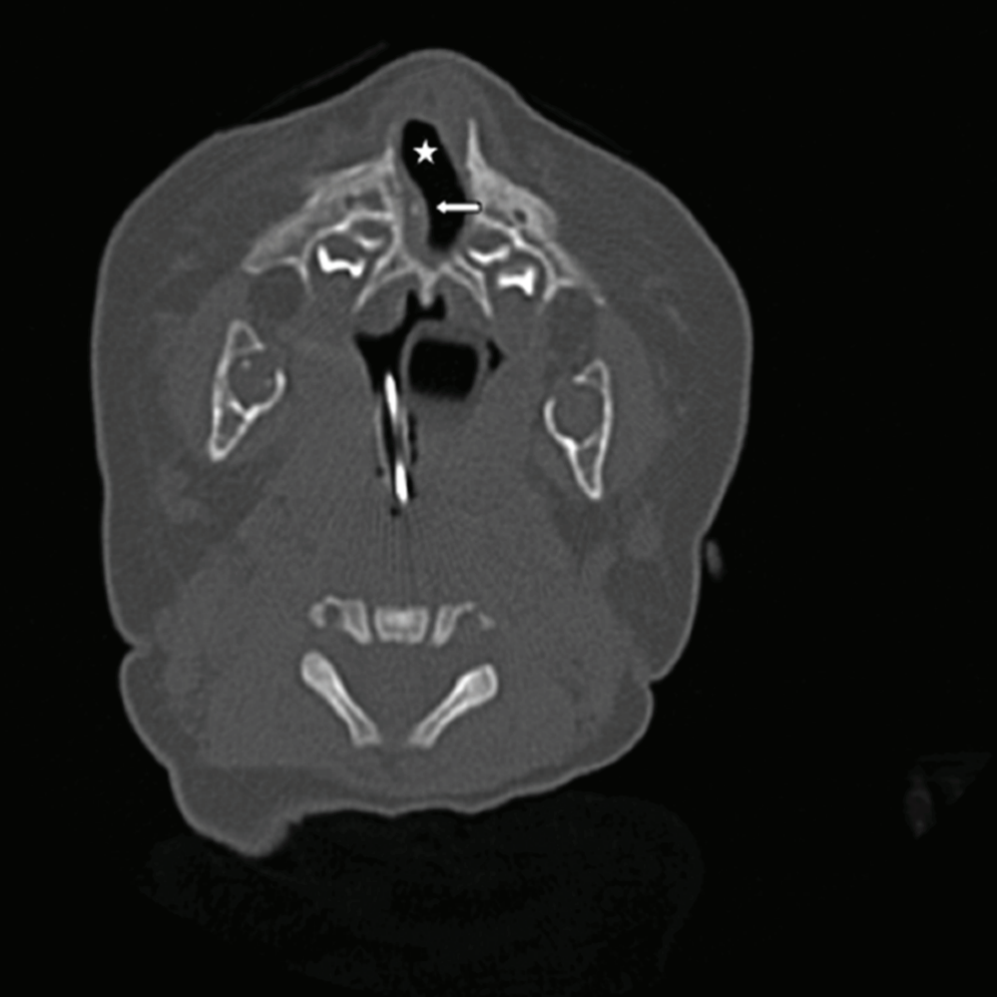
CT image (post-stenting): axial view of the widened pyriform aperture (star) measuring 10.3 mm. Nasal septum was partly eroded (white arrow).
